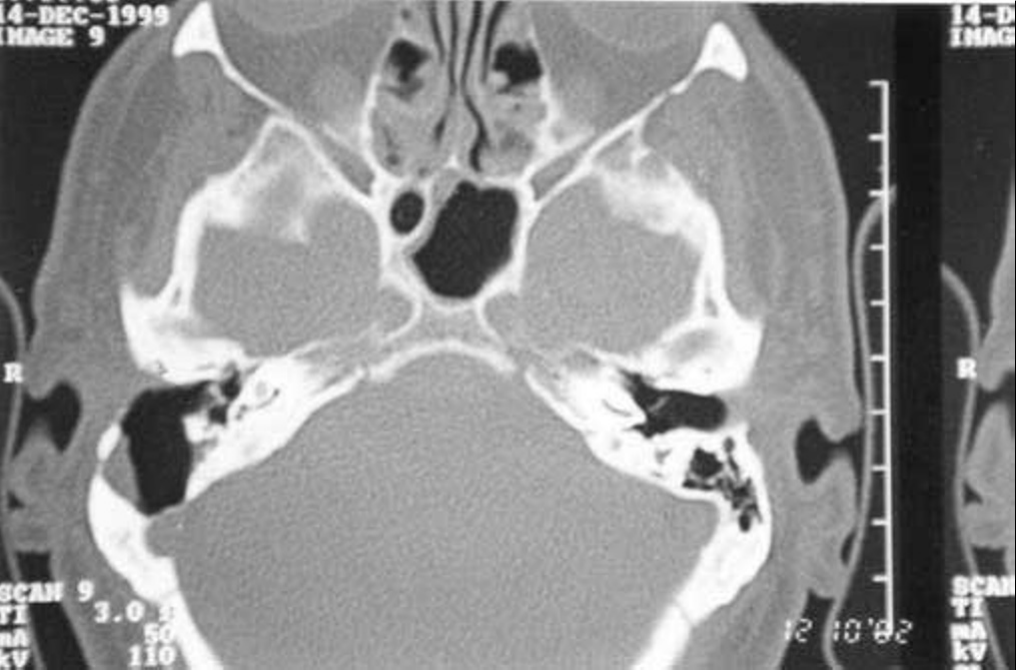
Temporal bone axial CT scan showing contact of the cholesteatoma's capsule with sigmoid sinus and meninges.
